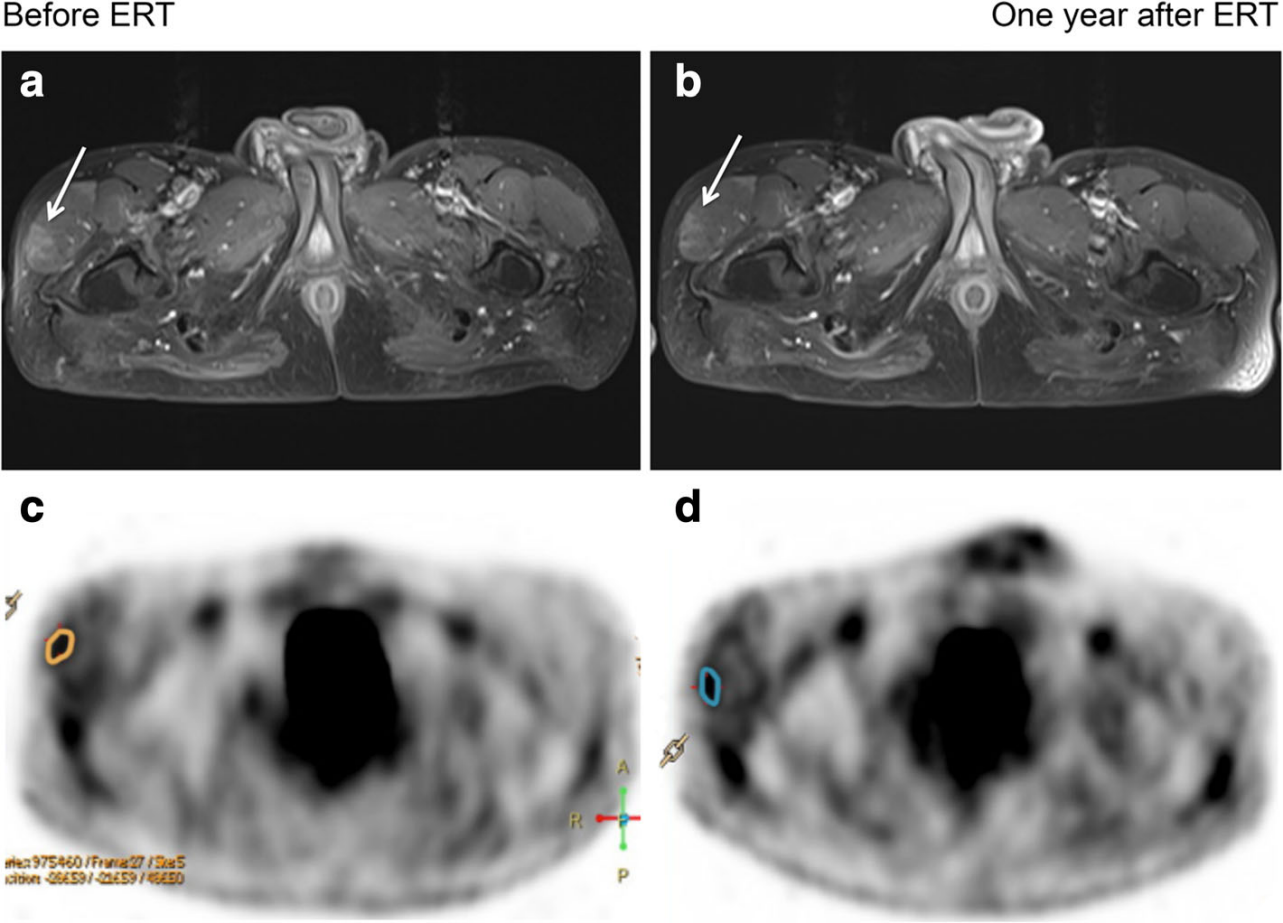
MRI (a, b) and FDG PET (c, d) before and after 1 year of ERT. MRI shows slight improvement, i.e., less hyperintensity of the tensor fasciae latae muscle (arrow) in the T1w sequence after gadolinium administration 1 year after ERT. FDG-PET show no significantly different tracer uptake in the region of interest (orange and blue lines) pre- and post-therapy
